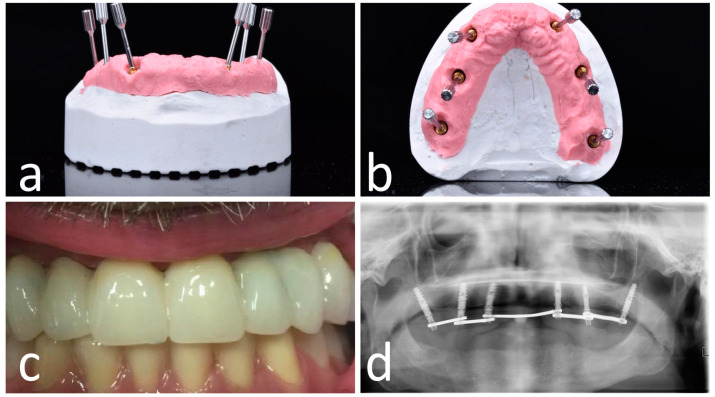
OT-bridge abutments use the “Extragrade™” feature, a system allowing to compensate up to 80° of the divergence between two implants (a,b). Pictures c and d show the clinical (c) and X-ray (d) view of the provisional prosthesis using OT-bridge abutments.
